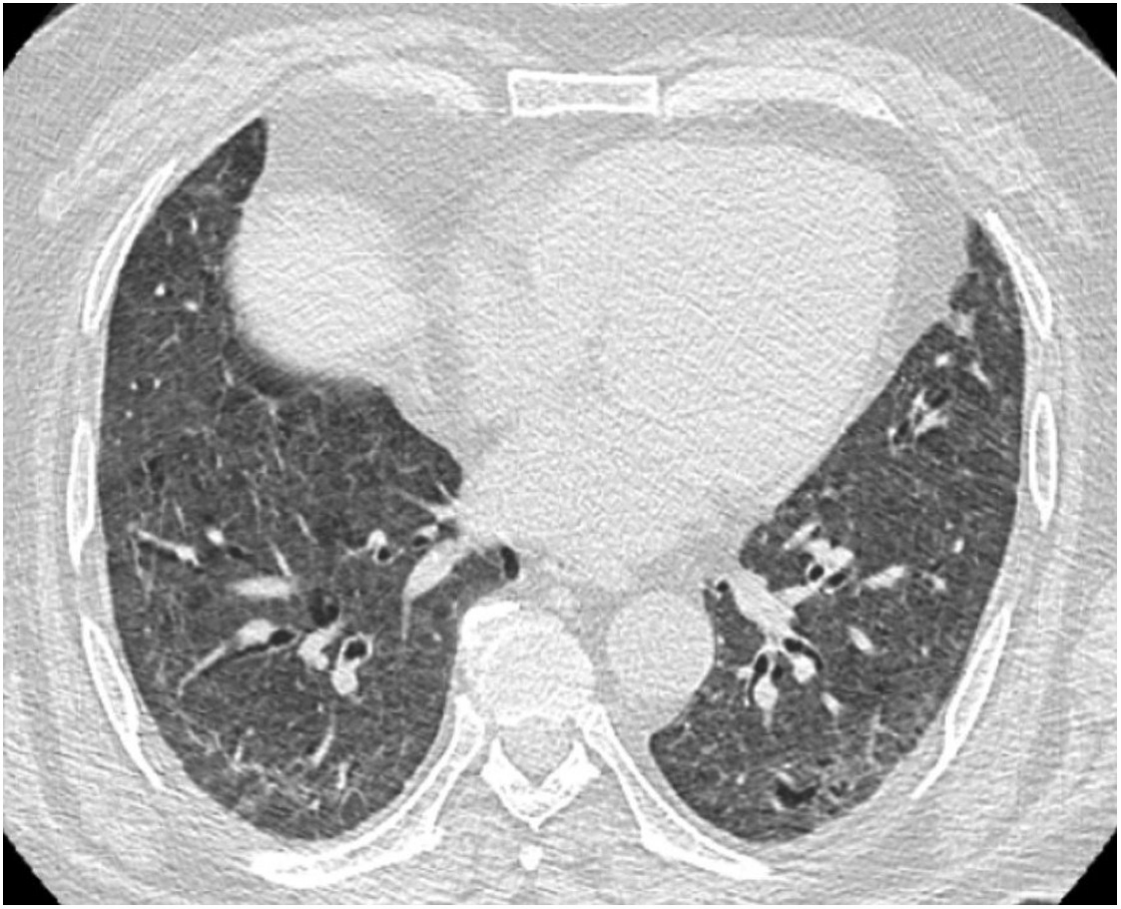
Reduction in lower lobe fibrosis at the second follow-up HRCT.
